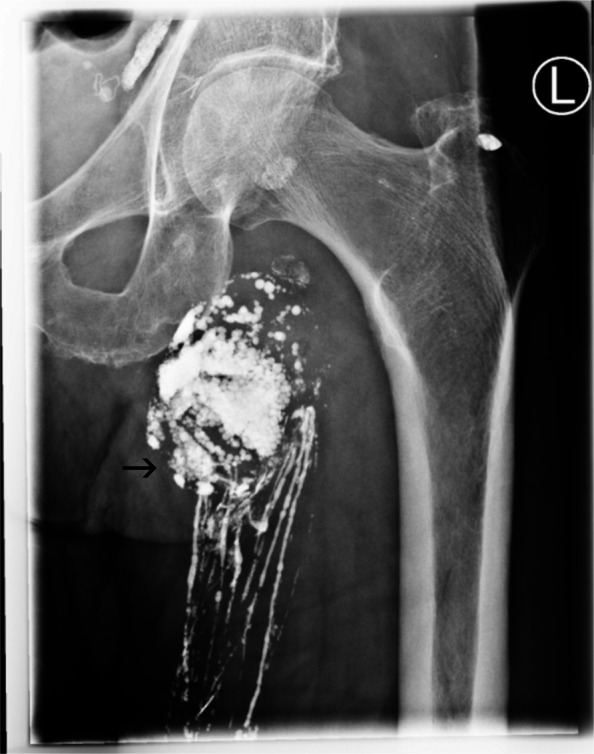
X-ray 24 h after intervention. An 82-year-old male patient with lymphatic fistula after inguinal lymphadenectomy. Documentation of the visualisation of the lymphatic fistula 24 h after the lymphangiography
